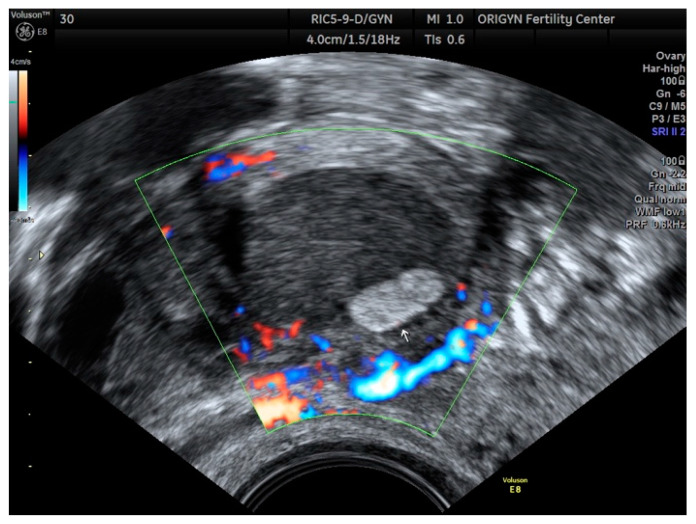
Ovarian endometrioma containing a hyperechoic area (white arrow) without Doppler signal.
